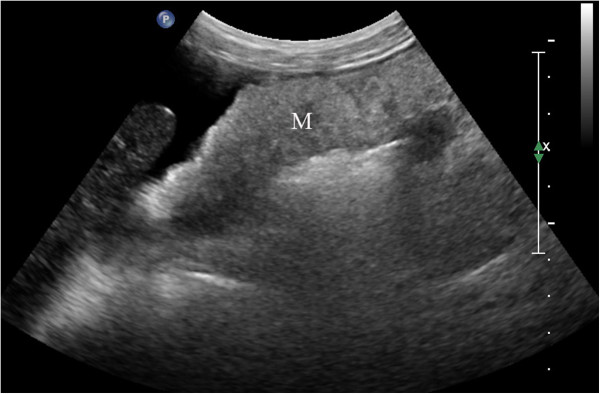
Data for a 56-year-old woman. Ultrasonography showed a cake-shaped thickening of the omentum and a nodosity-shaped thickening of the peritoneum (M); these thickenings were pathologically diagnosed as metastatic mucinous adenocarcinoma.
